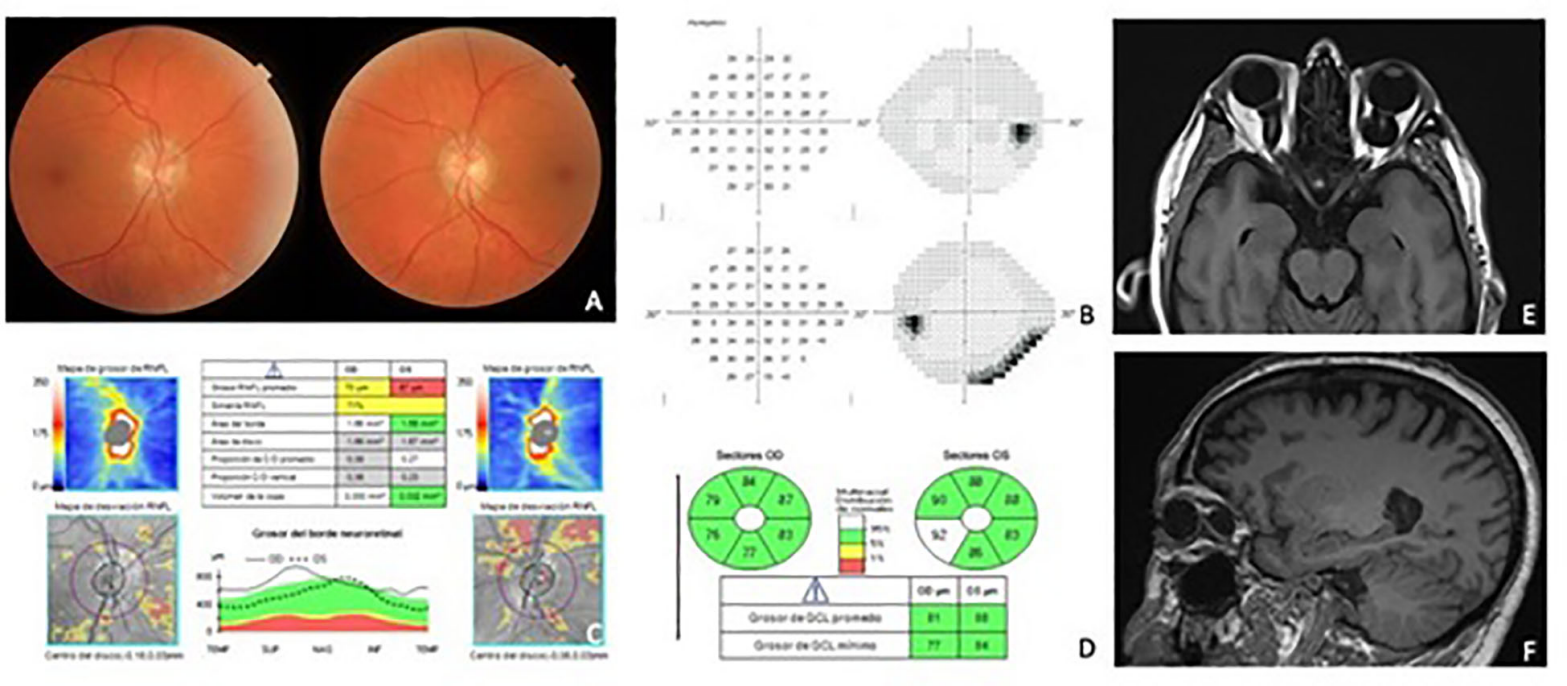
CASE 2 (images from 2023—3 years of follow-up showing no evidence of progression since the diagnosis). (A) Right and left eye fundoscopy. (B) 24-2 Visual field of both eyes with peripheral inferior nasal defect in the left eye. (C) ONH OCT scan. (D) GCL sectors of both eyes. (E) MRI axial view in T1 dark fluid with bilateral optic nerve sheath dilatation and optic nerve tortuosity. (F) Orbital MRI, sagittal view in T1 at the right orbit plane.
